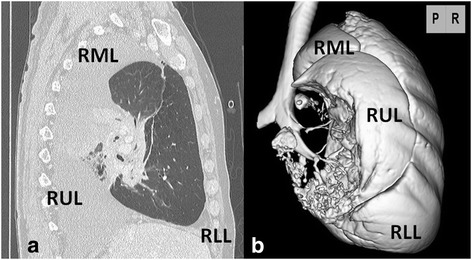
a Sagittal CT at the onset of torsion showed that the right upper lung lobe with the large tumor had rotated 90° counterclockwise toward the hilum. b Three-dimensional CT by the view of posterior showed that the middle and lower lung lobes had been pushed upward and forward respectively. The *blank space* showed a tumor. *RUL* right upper lobe, *RML* right middle lobe, *RLL* right lower lobe
